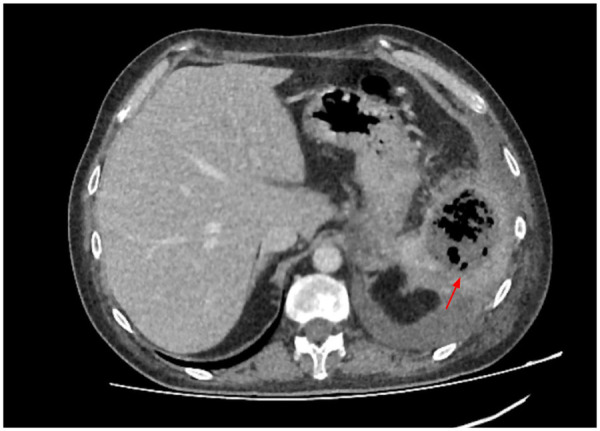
Contrast-enhanced CT of the abdomen. Red arrow—splenic abscess.
